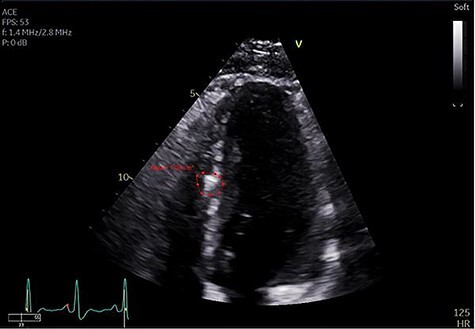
Echocardiogram identifying bullet fragment in the interventricular septum.
